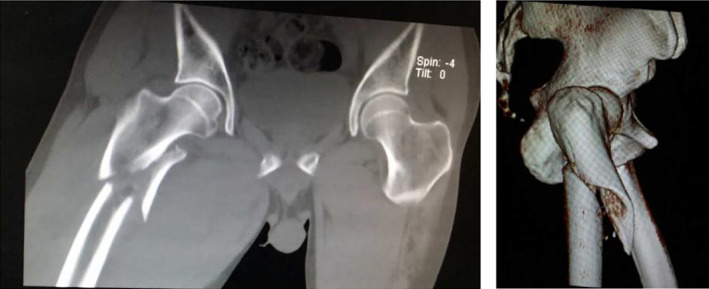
Preoperative CT and 3D scans.
